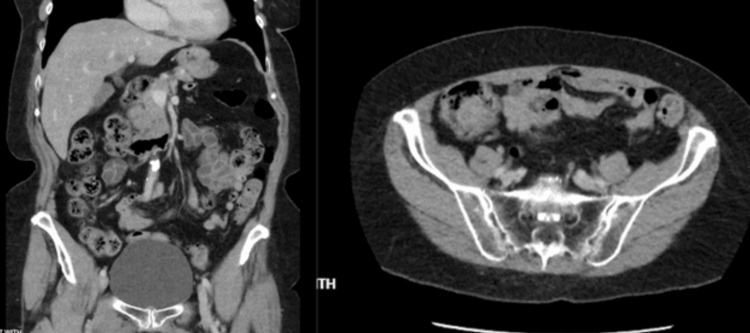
CT scans three months after completion of chemotherapy demonstrated no avid malignancy, including no presence of cecal mass.
